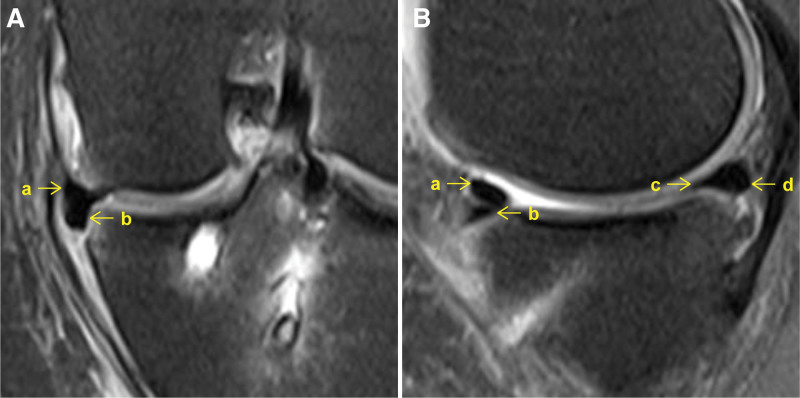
Postoperative coronal and sagittal magnetic resonance images. (A) a, remaining midbody; b, midbody tendon autograft. (B) a, anterior horn tendon autograft; b, remaining anterior horn; c, posterior horn tendon autograft; d, remaining posterior horn.
